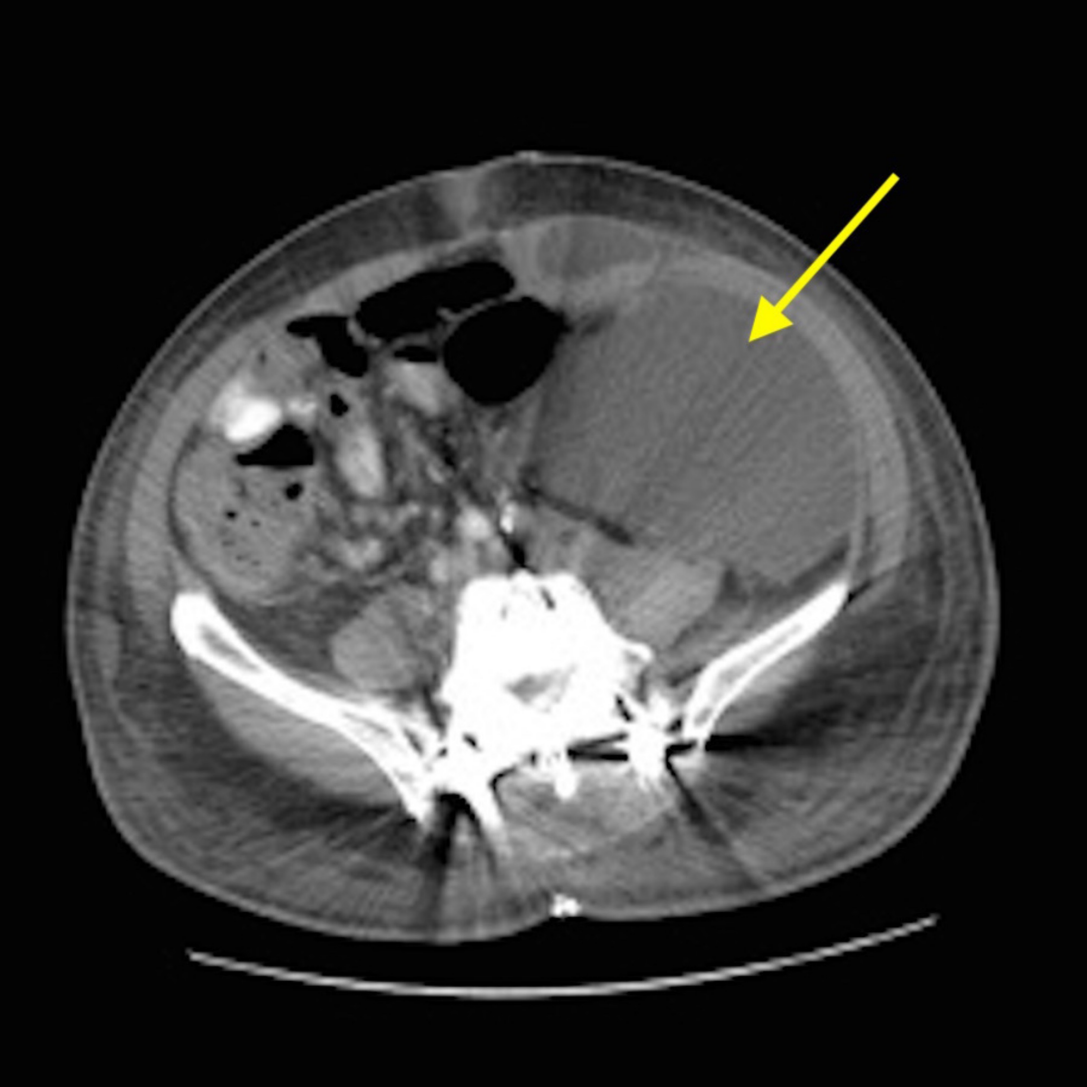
Computed tomography (CT) scan of the abdomen and pelvis. CT scan of the abdomen and pelvis demonstrating a large cystic mass in the retroperitoneal and pelvic cavities, displacing bowel rightward and compressing the left kidney.
